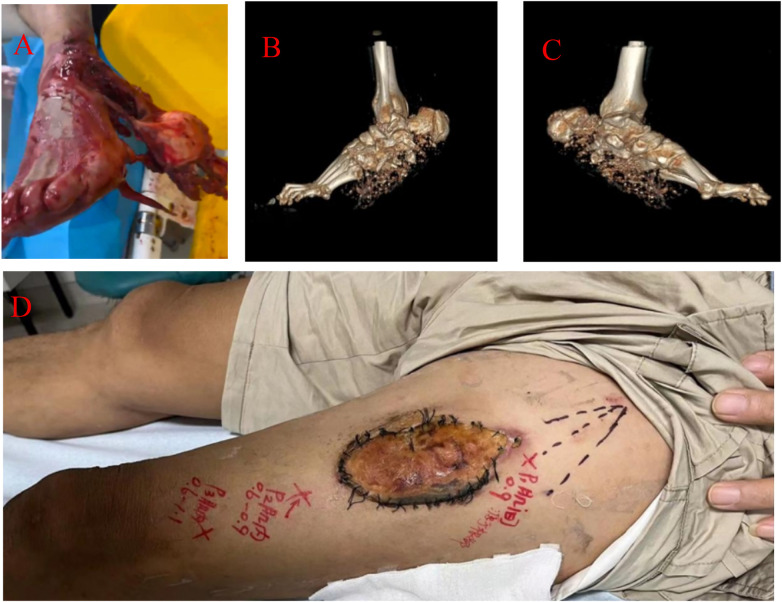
(A–C) preoperative presentation of the left foot and three-dimensional computed tomography imaging demonstrate a comminuted fracture of the calcaneus with extensive bone loss, alongside substantial soft-tissue defects involving the medial and lateral malleoli and the plantar region, with only a remnant of heel skin preserved. (D) Intraoperative view showing the temporary transfer and fixation of the preserved heel skin as a “parasitic” flap to the thigh.
